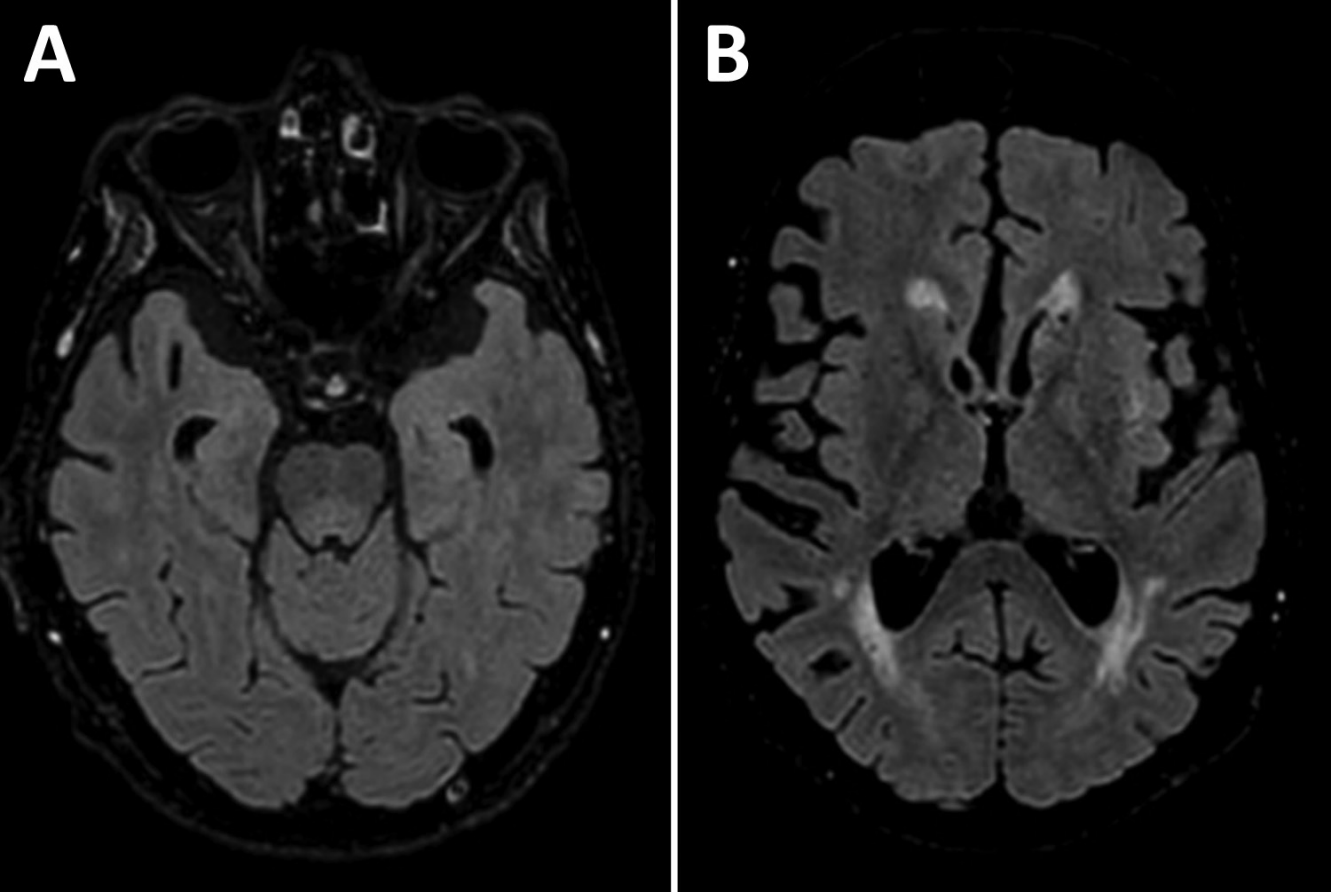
Axial FLAIR magnetic resonance imaging brain sequence in a patient with locally acquired Japanese encephalitis virus detected using clinical metagenomics, New South Wales, Australia. A) Equivocal hyperintensity in the dorsal midbrain and pons; B) sparing of the thalamus and basal ganglia.
